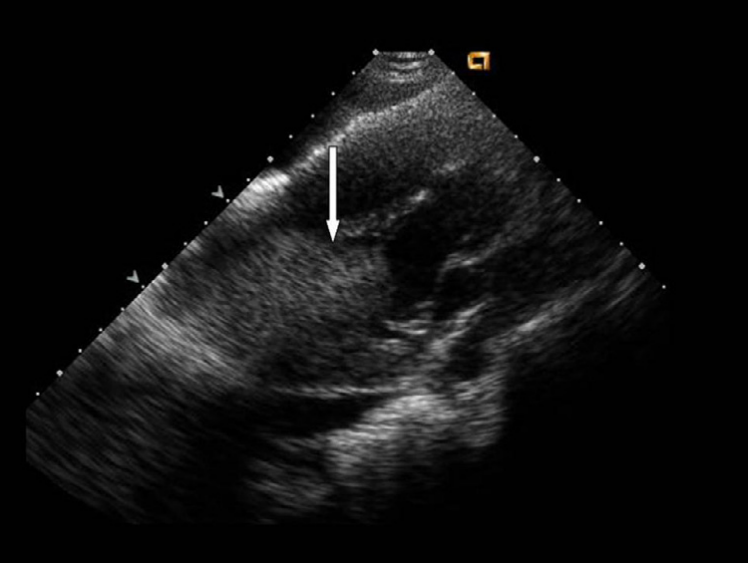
A silicone breast implant in this patient caused marked limitation of echocardiographic acoustic window and image acquisition in the parasternal long axis view obscuring left ventricular cavity and septum.
